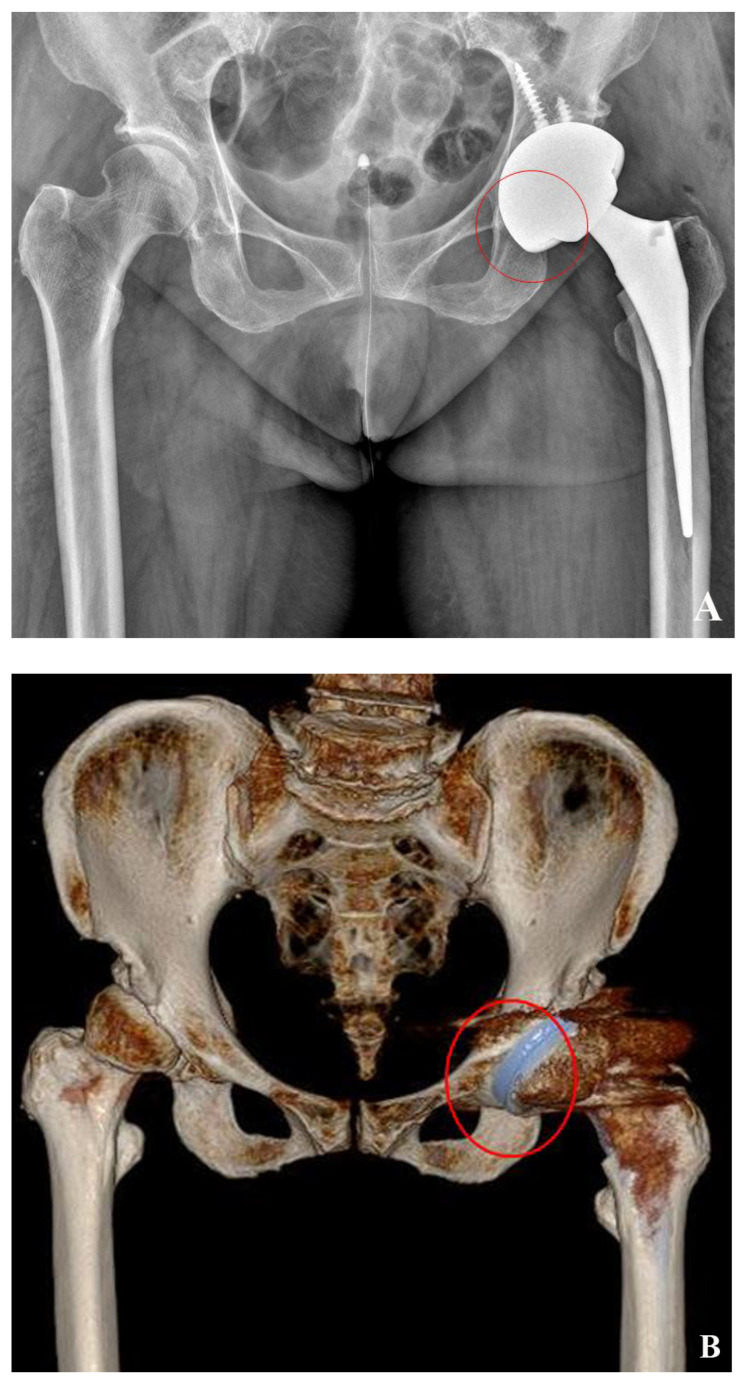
Differences of host bone coverage between plain radiograph and 3D CTs. Inferomedial side of acetabular cup (red circle) was calculated as covered surface in 2D-based host bone coverage measurement. (A) On the other hand, the lack of bone coverage on the inferomedial side of the cup (red circle) was detected in the 3D reconstruction of the CT scan (B).
